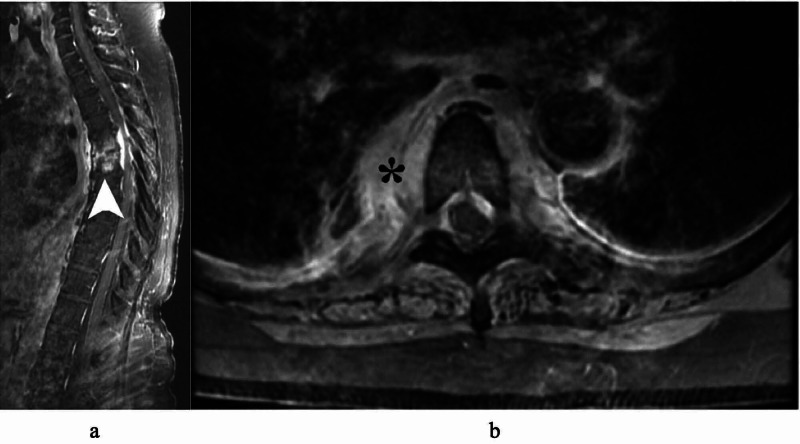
MRI thoracic spine T1 with contrast in sagittal (a) and axial (b) displaying T5-6 body and epidural enhancements (arrow) concerning for osteodiscitis and epidural abscess. There is paraspinal enhancement (asterisk) adjacent to the lung.
